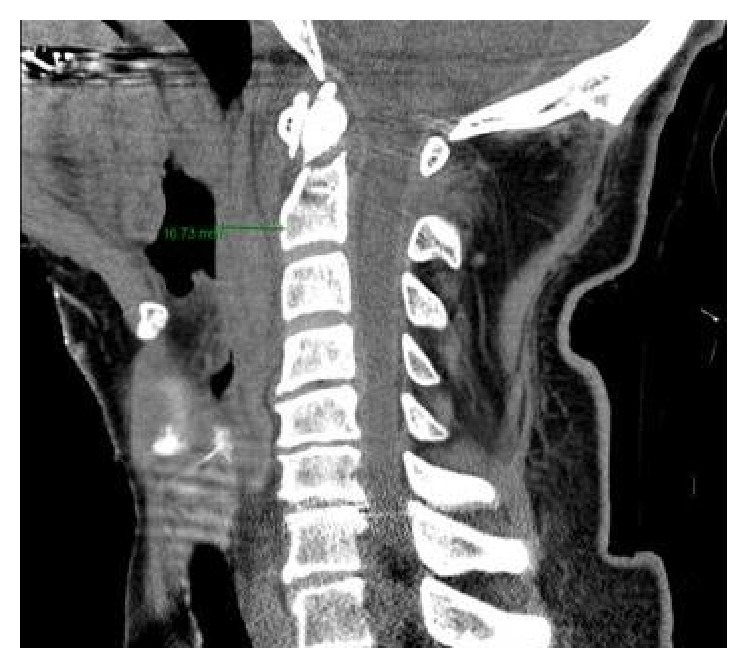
Midsagittal CT soft tissue window demonstrating prevertebral soft tissue swelling of 16.7 mm which is excessive for a transverse Type 2 dens fracture.
